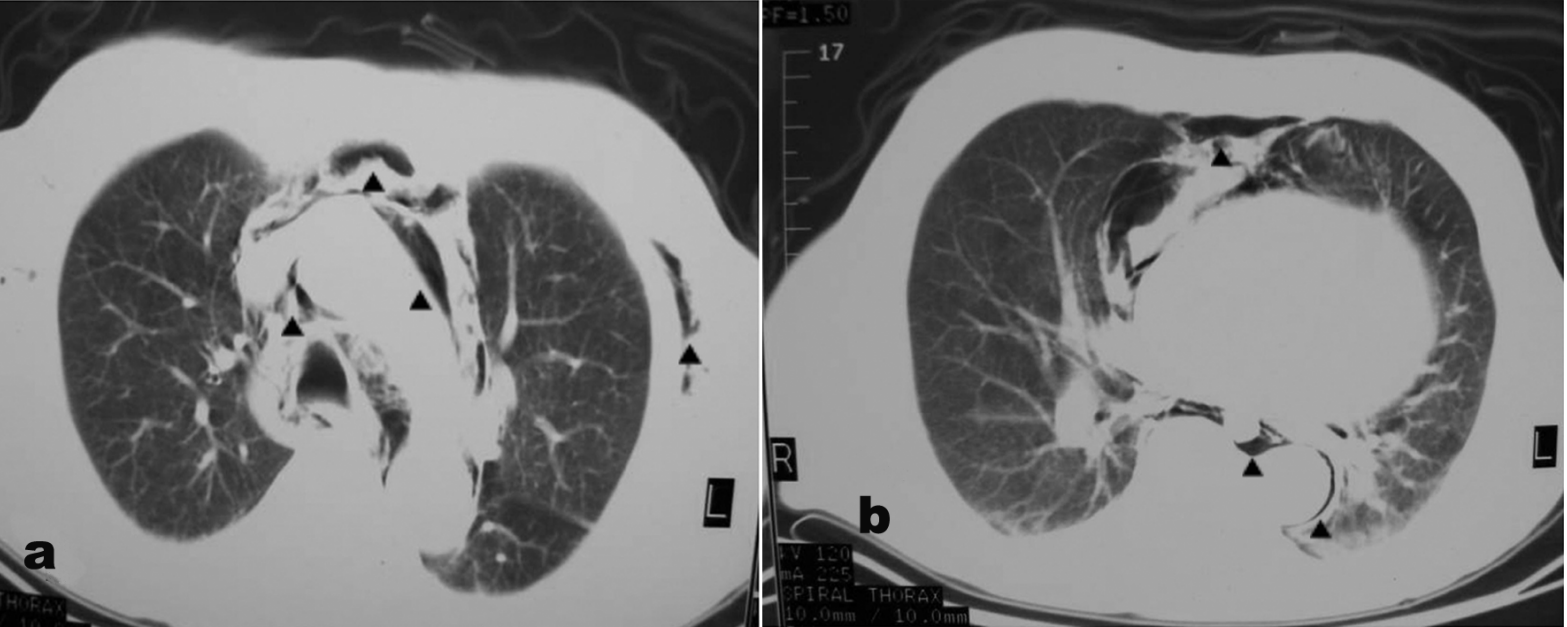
Computed tomography of the chest indicating the presence of subcutaneous emphysema and pneumomediastinum (arrows).
